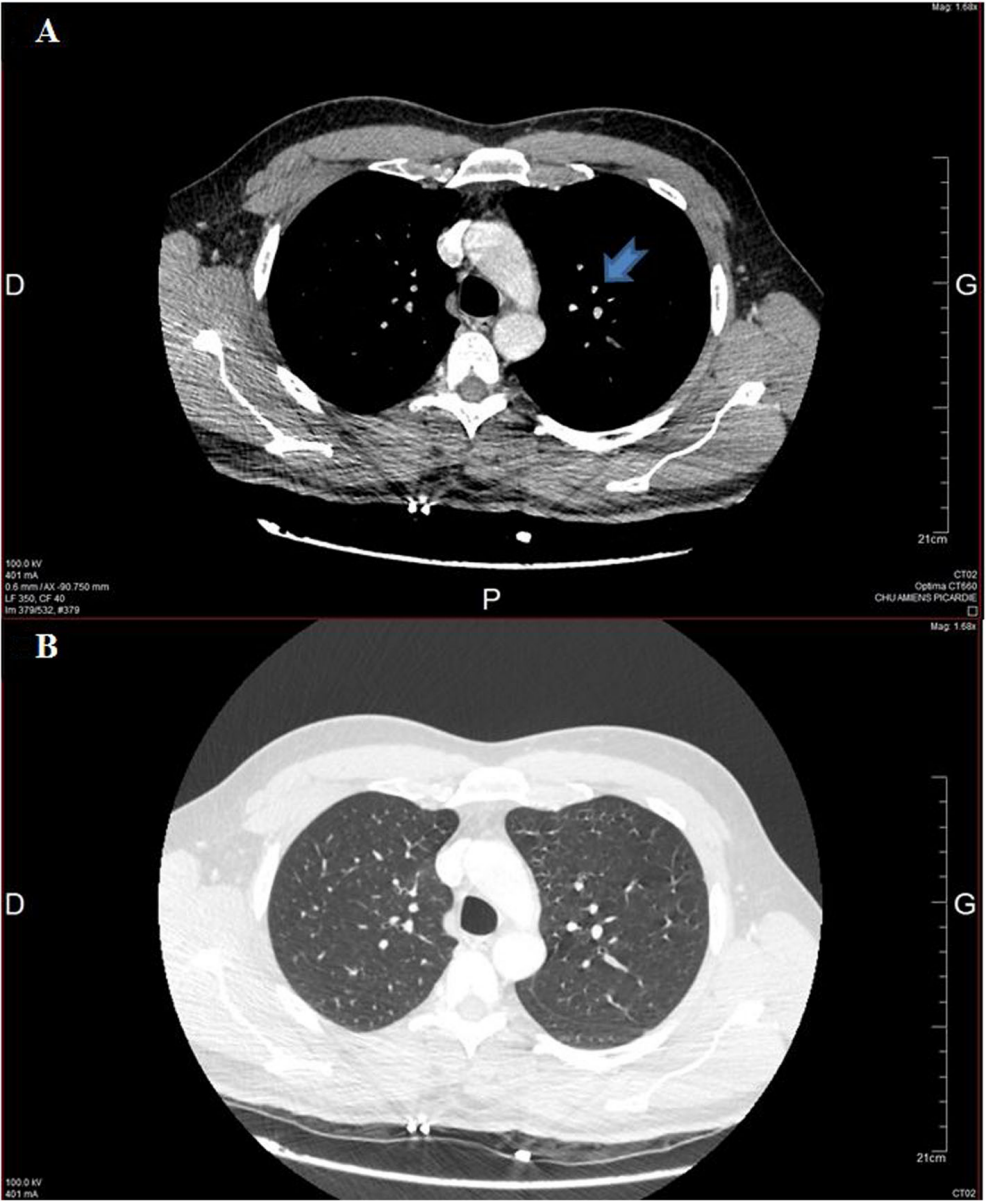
Transverse section CT scan. a: Mediastinal window showing a possible left superior lobar sub-segmental defect (arrow), subject to a poor quality of the contrast injection. b: Corresponding parenchymal window. Note the absence of lung parenchymal consolidation or ground-glass opacities
